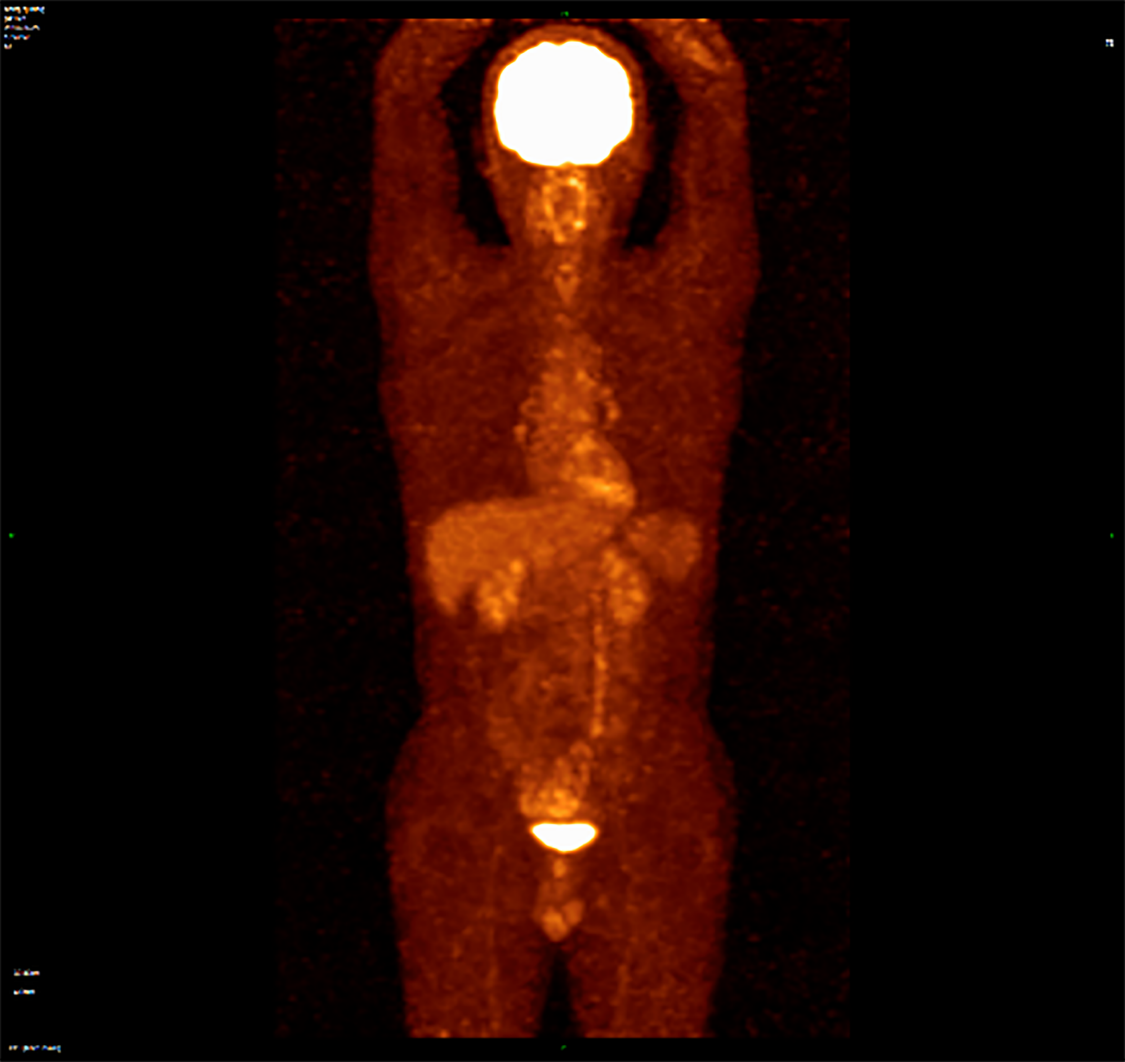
PET/CT did not show focal hypermetabolism of those lesions corresponding to the nodules in CT.
